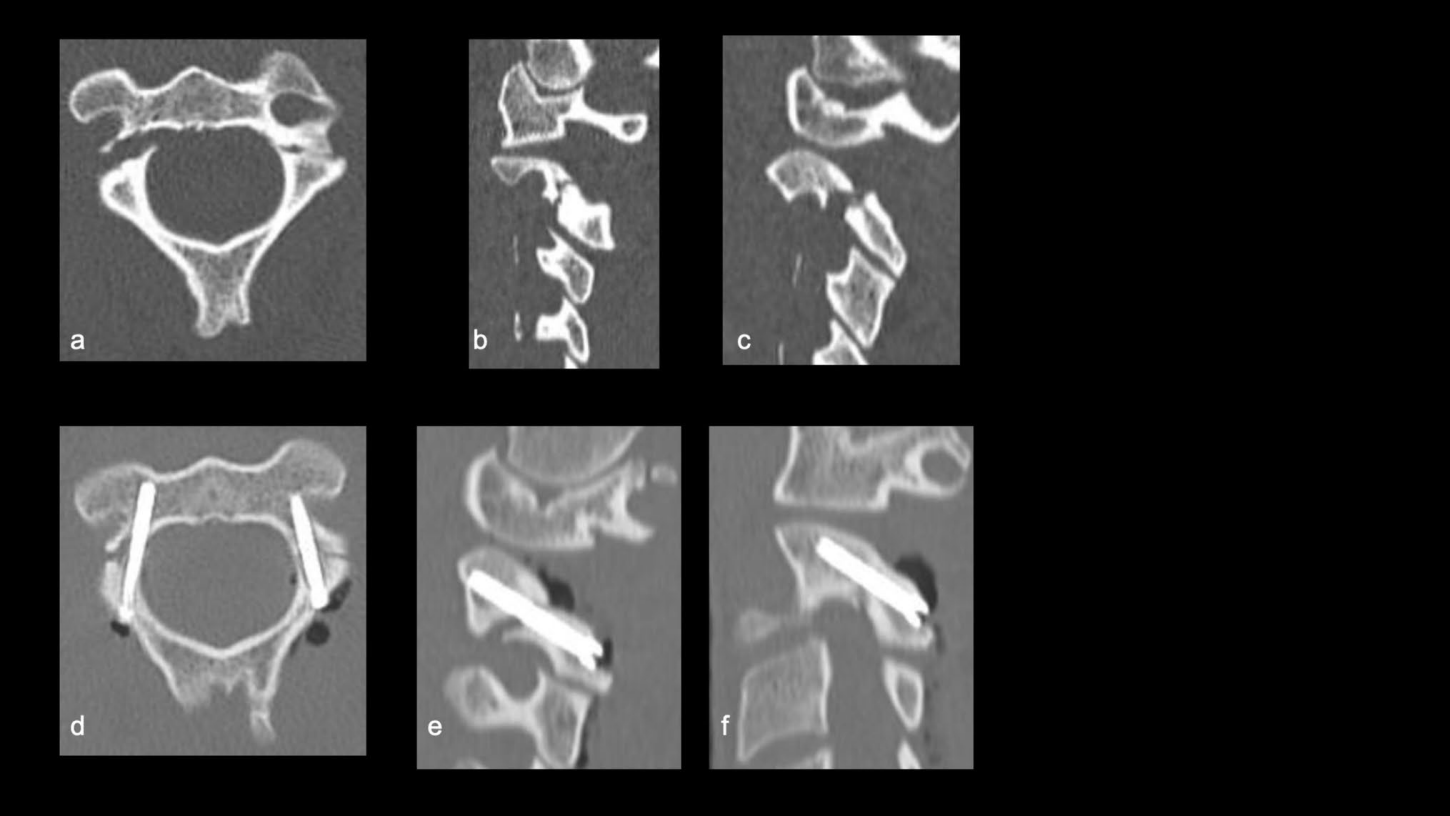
Preoperative axial (a) and sagittal (b, c) cervical spine CT scan showing a fracture of the two pedicles of C2. Postoperative axial (d) and sagittal (e, f) cervical spine CT images demonstrating C2 transpedicular screws (Judet technique) with reduction of the fracture gap
